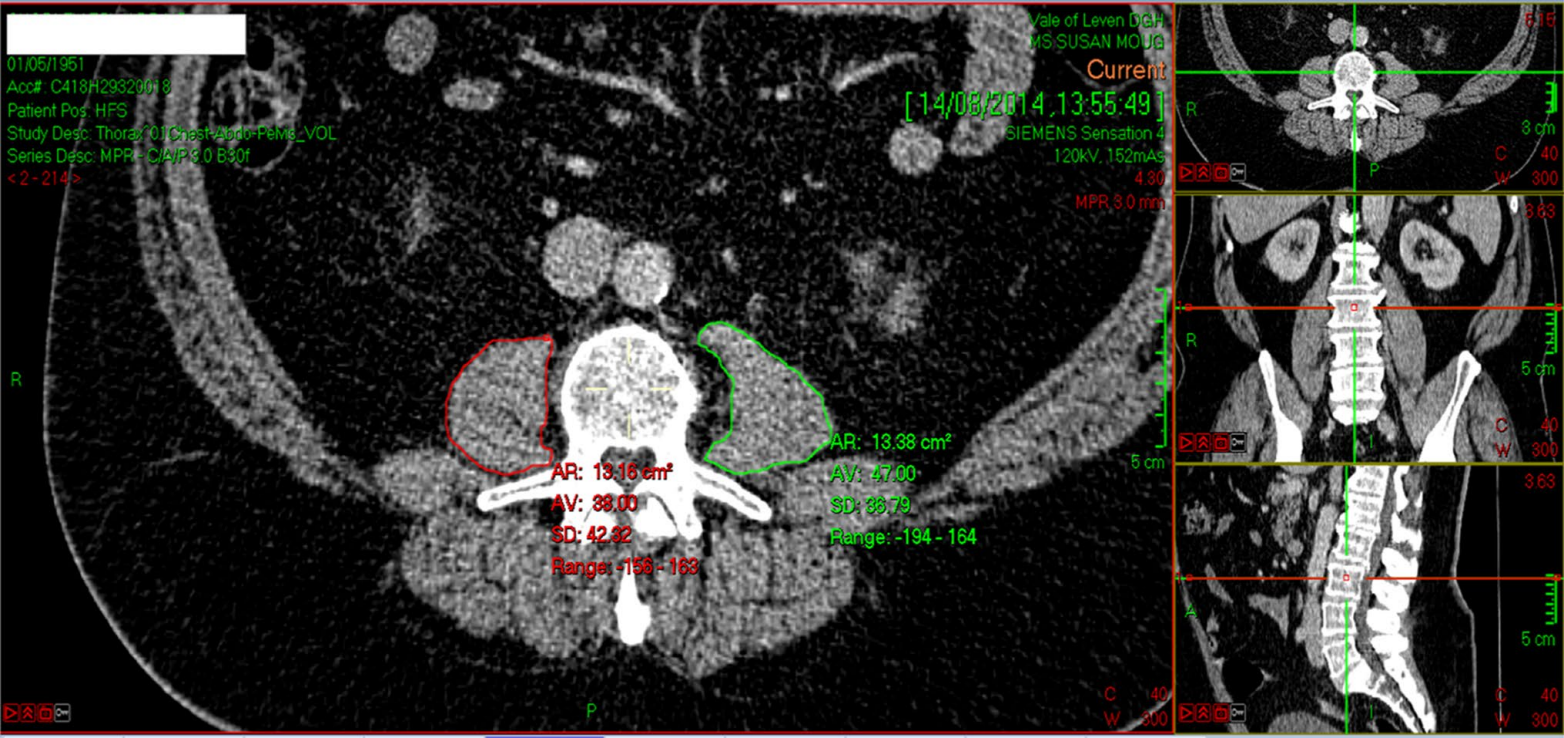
CT image at the level of the L3 vertebra, demonstrating the method of outlining the left (green) and right (red) psoas muscle and measuring their respective areas
